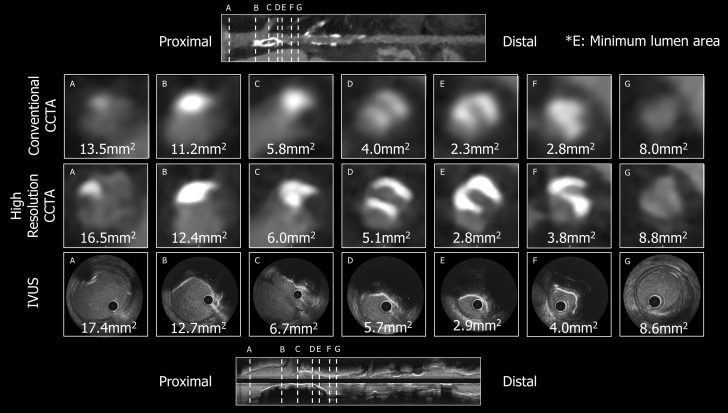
Comparison of Lumen Area and Calcified Plaque by Imaging Modalities Comparison of lumen area and calcified plaque assessment between conventional CCTA, AI-enhanced CCTA, and IVUS. AI-enhanced CCTA provided lumen measurements closer to IVUS (2.8 vs 2.9 mm^2^) compared with conventional CCTA (2.3 mm^2^) and depicted calcified plaque more clearly. AI = artificial intelligence; CCTA = coronary computed tomography angiography; IVUS = intravascular ultrasound.
